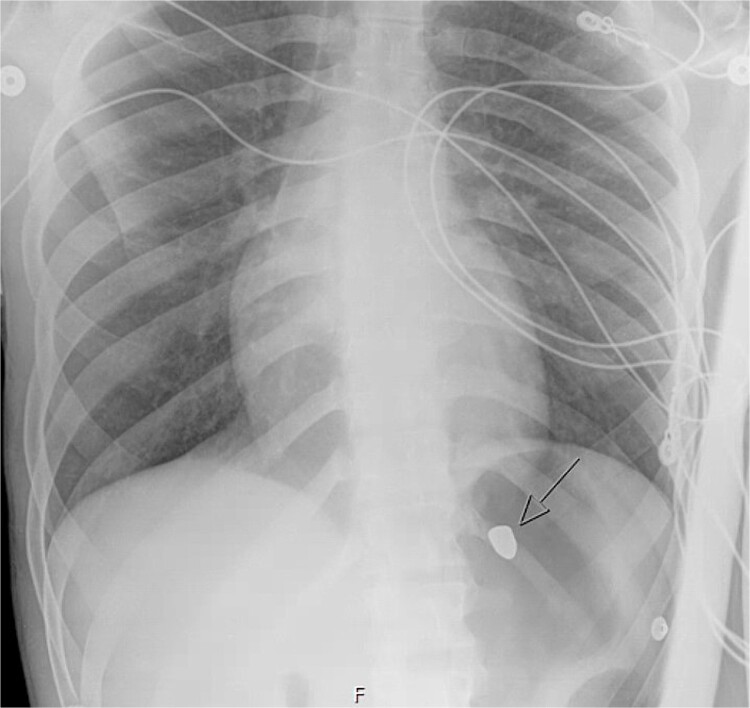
Hospital day 1 chest X-ray.
